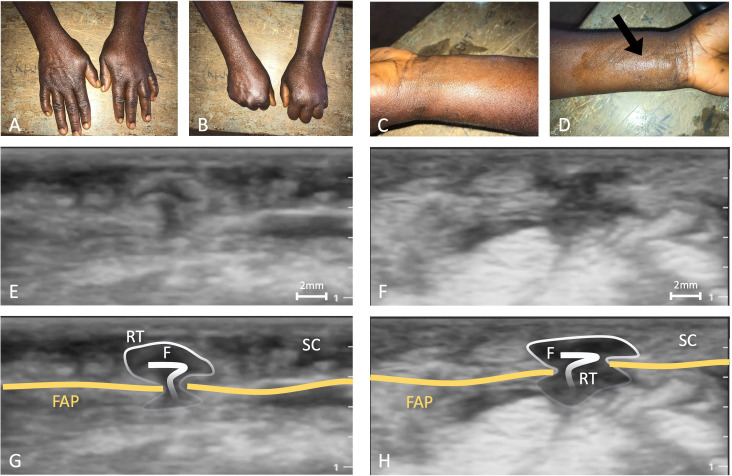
Findings of patient 17: A + B) typical Calabar swelling on the left hand: both hands in an expanded position (A) and with fists closed (B); C + D) subcutaneous nodule on the left palmar forearm, indicated by black arrow (D); E + F) the corresponding ultrasound images showing the subcutaneous nodule presenting as a retention in the ultrasound, with trans-fascial gap and hyperechoic structure inside in longitudinal (E) and transversal scan (F) (both show still images from S5 Video at seconds 3, respectively); and G + H) Corresponding ultrasound images with annotations. Legend: RT = retention; F = filaria; SC = subcutaneous tissue; FAP = *palmar antebrachial fascia* (orange); FDS = *flexor digitorum superficialis muscle*. Pictures A to D were taken by LV.
